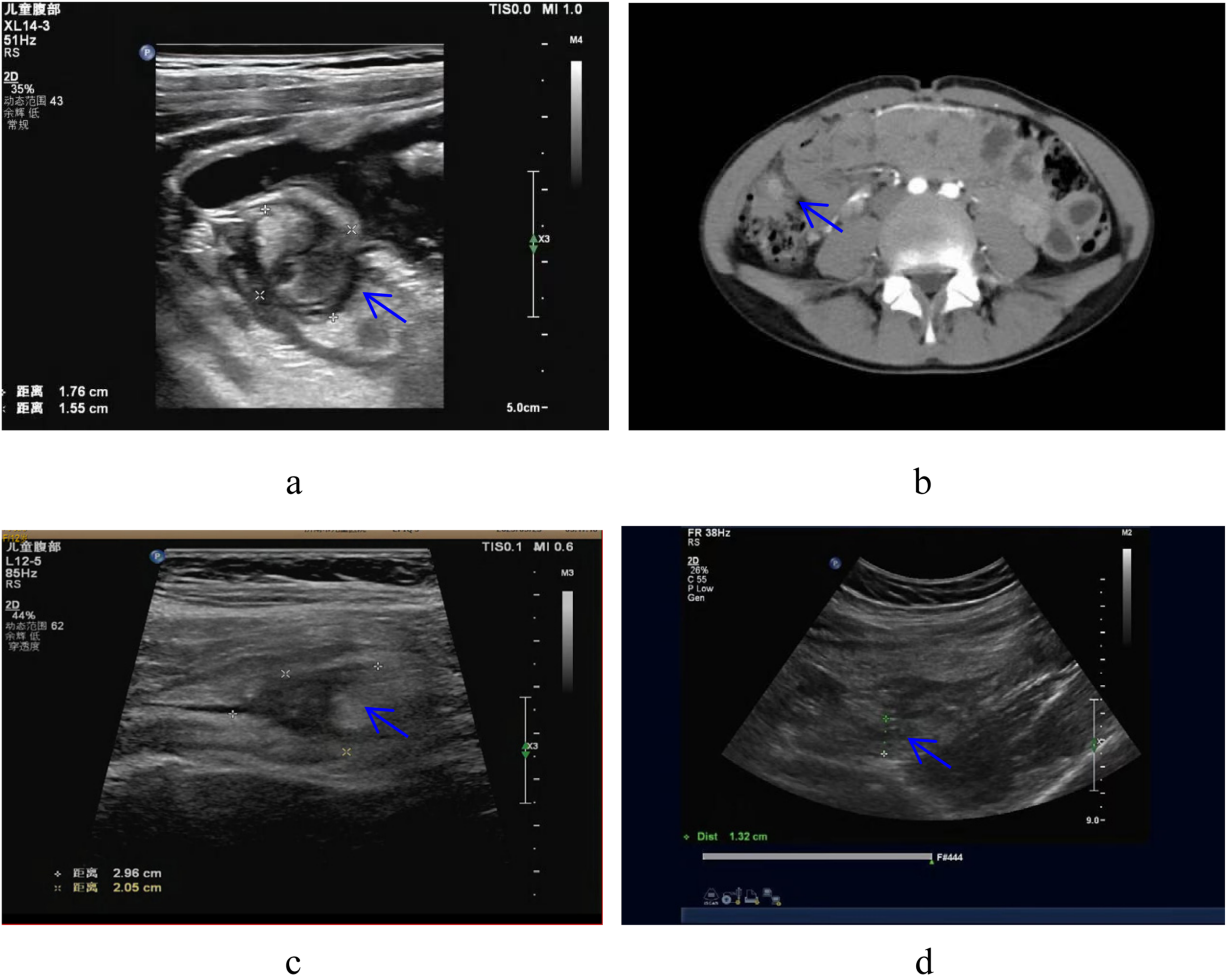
(a,b) in children with simple cecal diverticulitis, inflammatory mass can be seen under ultrasound and CT (→ mark). (c,d) In children with complex cecal diverticulitis was misdiagnosed as acute appendicitis under ultrasound. Ultrasound and CT scans follow standardized protocols and are observed jointly by two senior specialists. When there is disagreement, the differences between observers are discussed through consultation to minimize differences between observers.
